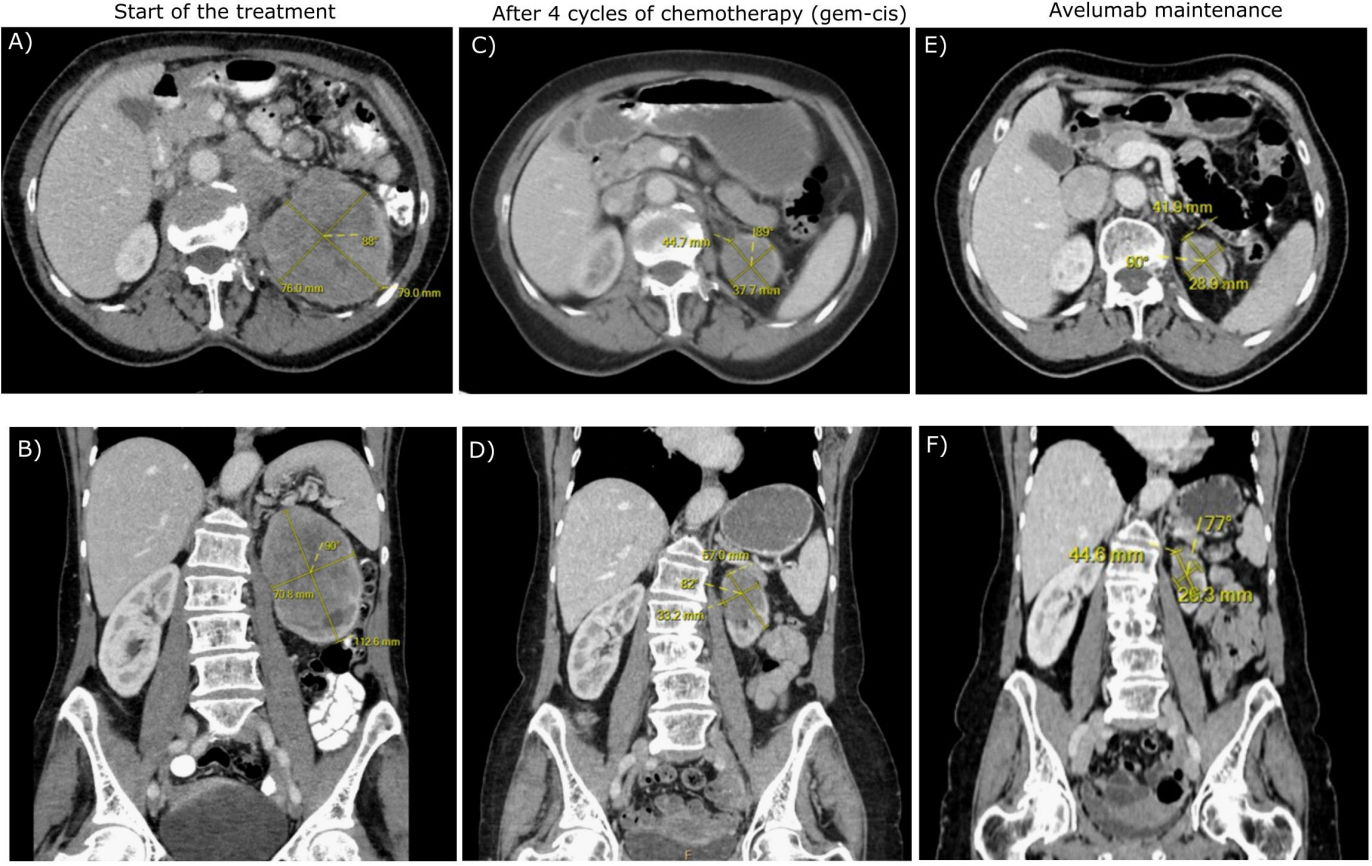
The transverse (A, C, E) and frontal (B, D, F) CT scans of the patient during the treatment.
